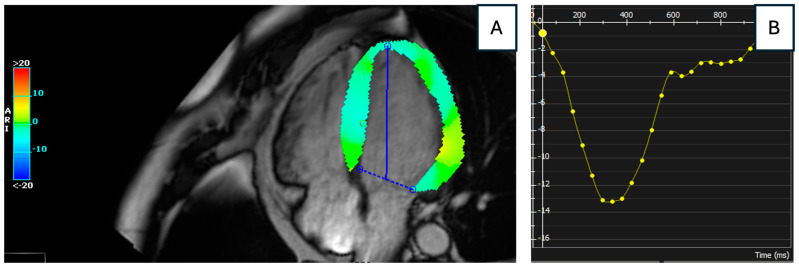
Mildly reduced left ventricular global longitudinal strain measured by cardiac magnetic resonance imaging. (A) depicts cardiac magnetic resonance feature tracking global longitudinal strain in four chamber view; (B) depicts left ventricular global longitudinal strain (y-axis) over time during cardiac cycle (x-axis). The peak negative value (–13.2%) indicates a reduced maximal degree of myocardial shortening during systole, suggesting impaired left ventricular systolic function.
